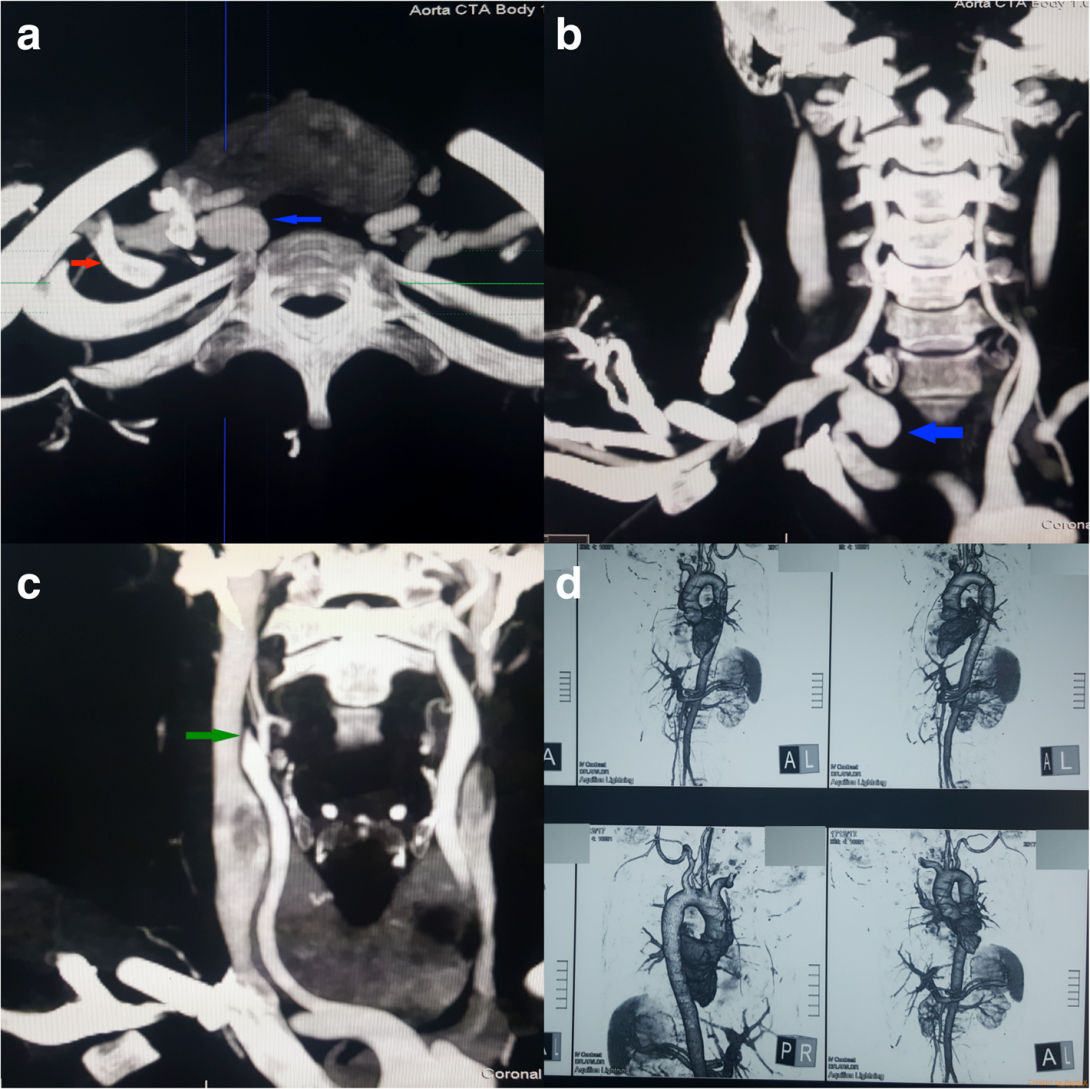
CT angiogram showing aneurismal dilatation (20 × 11 mm) of the first part of the right subclavian artery(in a and b shown by *blue arrow*). Tapering of the distal part of the right carotid bulb at the origin of the right internal carotid artery (shown by the *green arrow*) was suggestive of right internal carotid artery dissection with thrombosis. *Red arrow* showing the cervical rib. Rest of the angiogram was normal (Shown by d)
